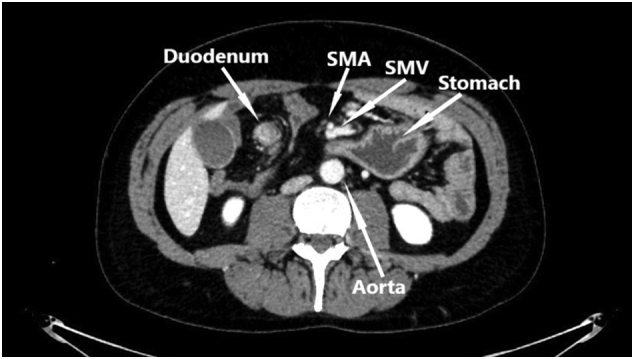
Preoperative contrast enhanced CT study image. Herniation of the mesentery through the lesser omentum.
